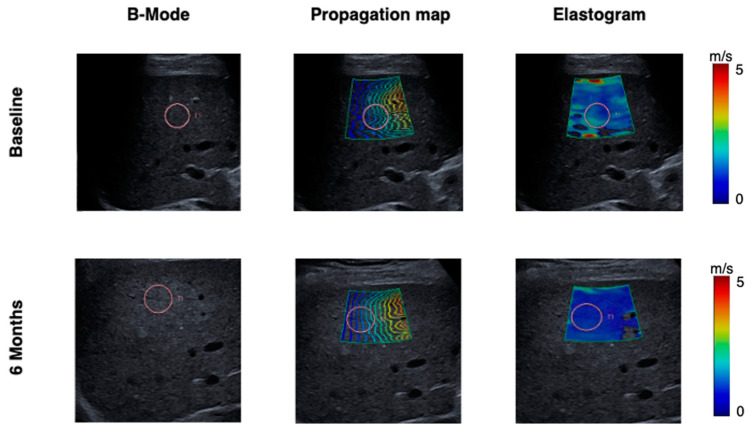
Representative ultrasound and shear wave elastography images at baseline and after 6 months (EOT+3). Shear wave speed decreased after treatment with direct-acting antivirals. The visualization of shear wave propagation in real-time allowed identification of areas with sufficient dynamic strain for precise elasticity reconstruction. The presence of a consistent and parallel wavefront pattern was considered indicative of a technically successful elastography examination.
